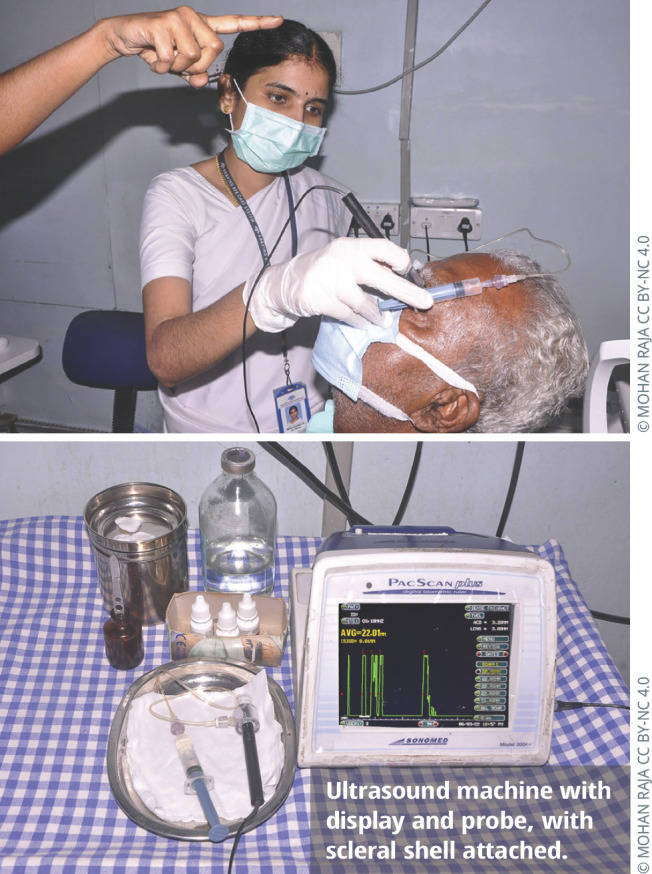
A biometrist performs immersion ultrasound biometry. INDIA
